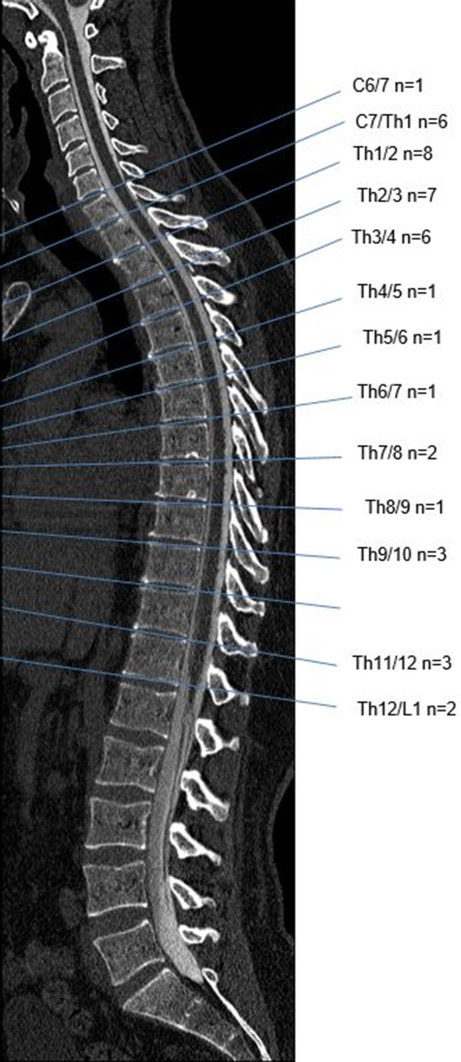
Location of 42 ventral leaks: 27 (64%) were in the upper thoracic spine. Prone dynamic CT myelography additional to dynamic subtraction myelography was performed in eight patients: five leaks were located at C7/Th1–Th2/3 (not highlighted separately in this Figure)
